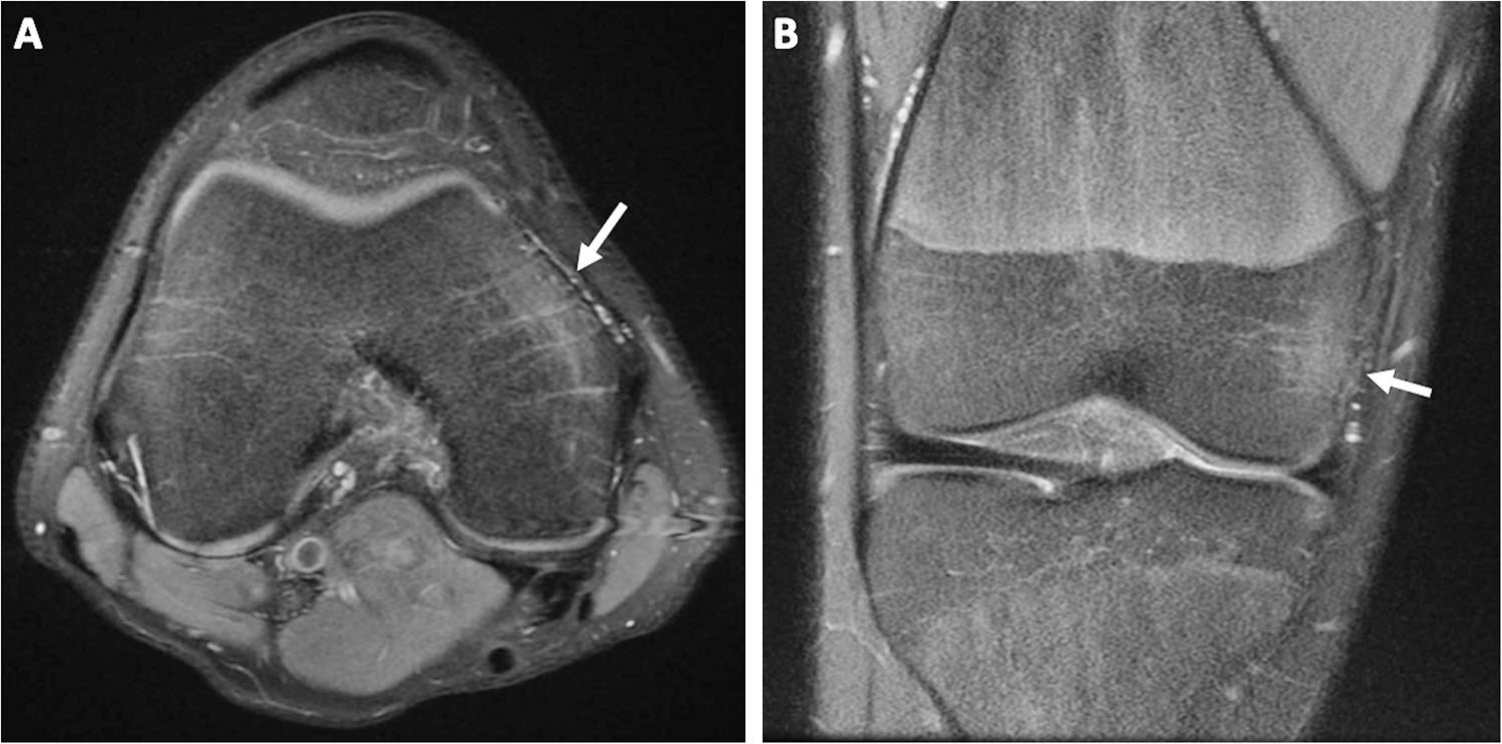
Magnetic resonance imaging of the right knee at 15 years of age. Axial (a) and coronal (b) proton density fat-saturated sequences again demonstrate marrow edema involving the anterior aspect of the medial femoral condyle (*arrows*)
